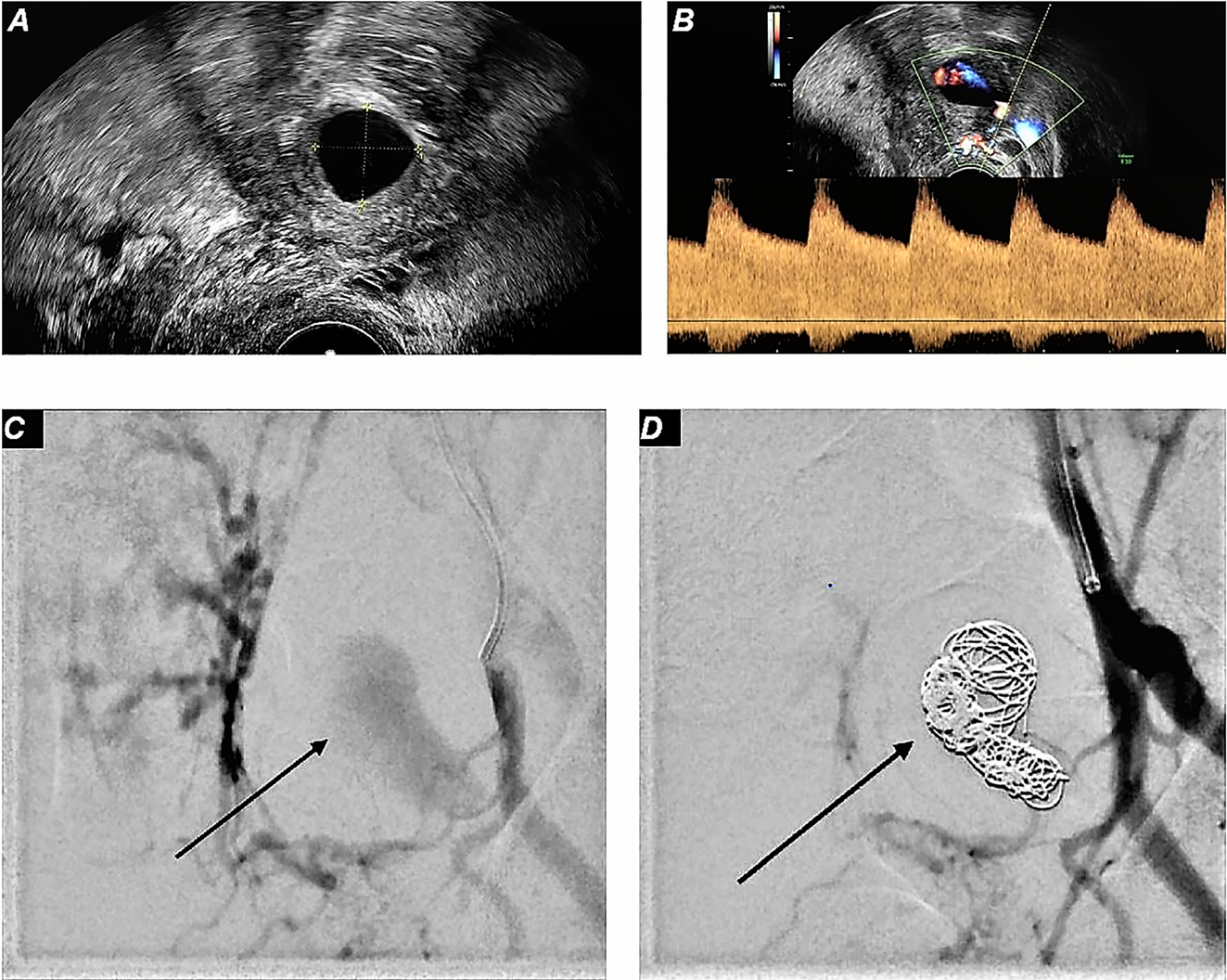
Uterine artery pseudoaneurysm. (A) Transvaginal ultrasound showing an anechoic lesion on the left side, suspicious for uterine artery pseudoaneurysm. (B) Transabdominal color Doppler ultrasound and pulsed wave showing high blood flow in anechoic lesion during systole coming from left uterine artery, suspicious for uterine artery pseudoaneurysm. (C) Digital subtraction angiography image of left uterine artery showing pseudoaneurysm filling with contrast medium. (D) Digital subtraction angiography image of left uterine artery showing occluded pseudoaneurysm after interventional radiological coil embolization.
